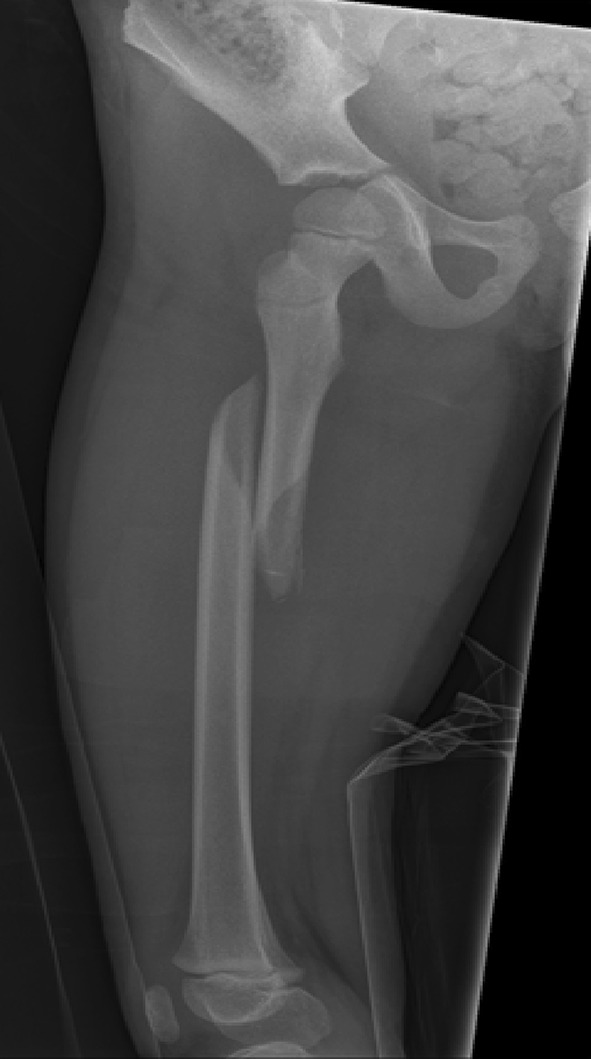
AP radiograph demonstrating a spiral femoral fracture ideal for treatment with flexible nails
